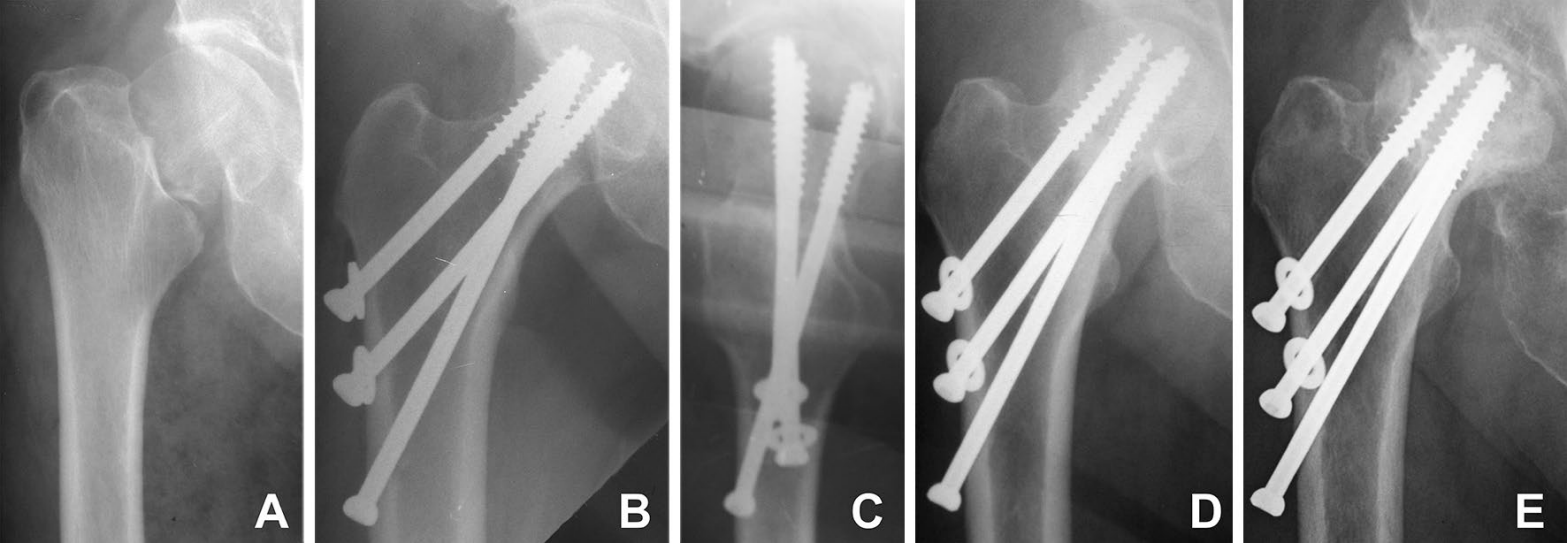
Exemplified X-rays of a 79 yo female patient with late AVN post bone union after BDSF treatment: AP view diagnostics (a), postoperative AP view (b), postoperative lateral view (c), AP view 10-month follow-up with bone union (d) and 24-month follow-up with late AVN (e)
